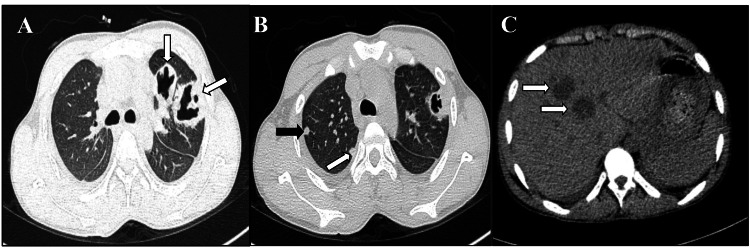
High-resolution computed tomography (HRCT) thorax (axial lung window) showing (A) areas of cavitatory consolidation in left upper lobe (white arrows) (B) few random and subpleural nodules in right upper lobe (white and black arrows) (C) Contrast-enhanced computed tomography (CECT) (upper abdomen) showing well defined homogeneous hypodense lesions in liver (white arrows)
